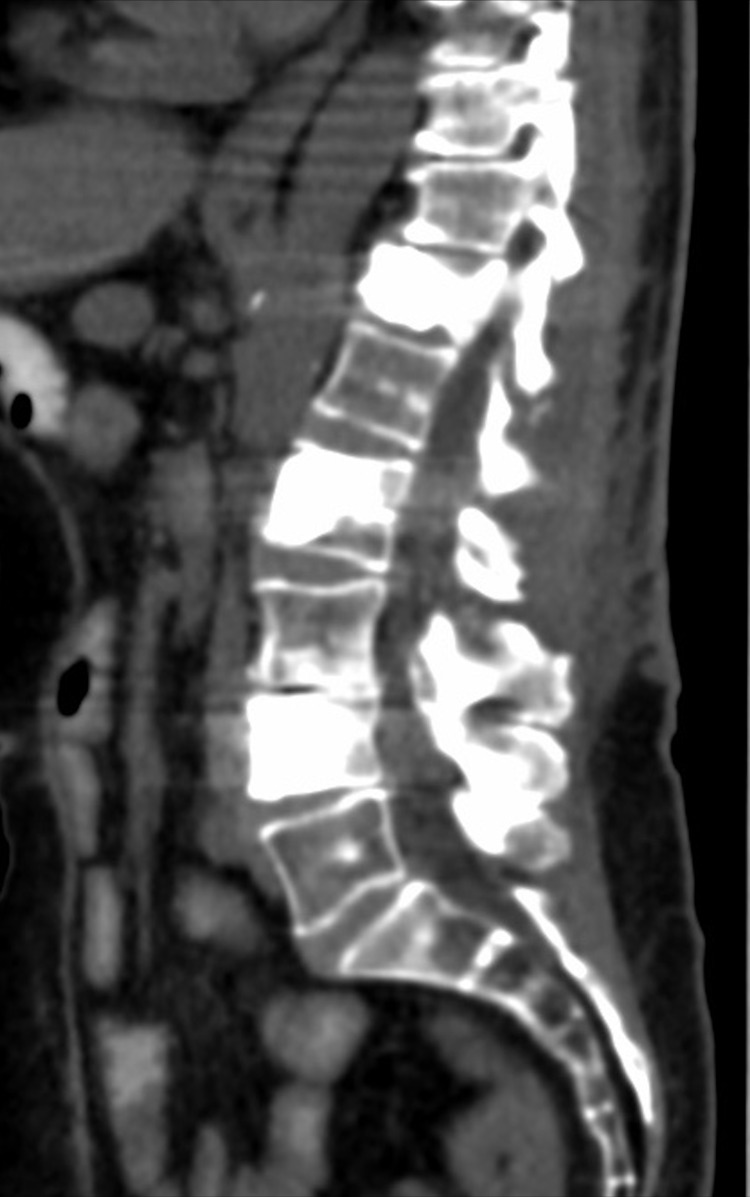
Postoperative CT at three-month follow-up Postoperative CT status post removal of hardware and kyphoplasty at T12 during three-month follow-up.
